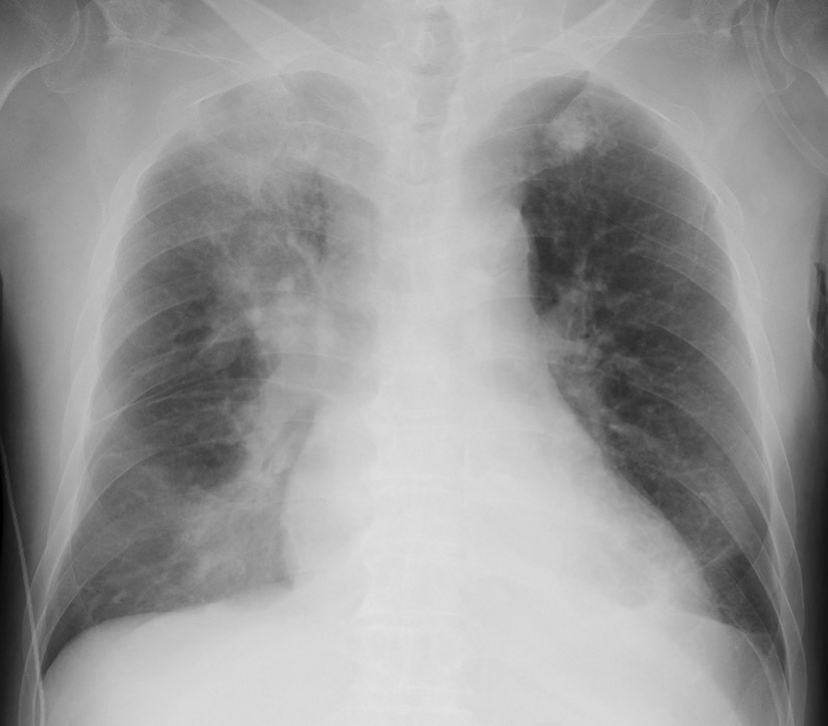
Chest X-ray on admission. Pulmonary consolidation on the right upper and lower lung of the patient, a 69-year-old Japanese male
